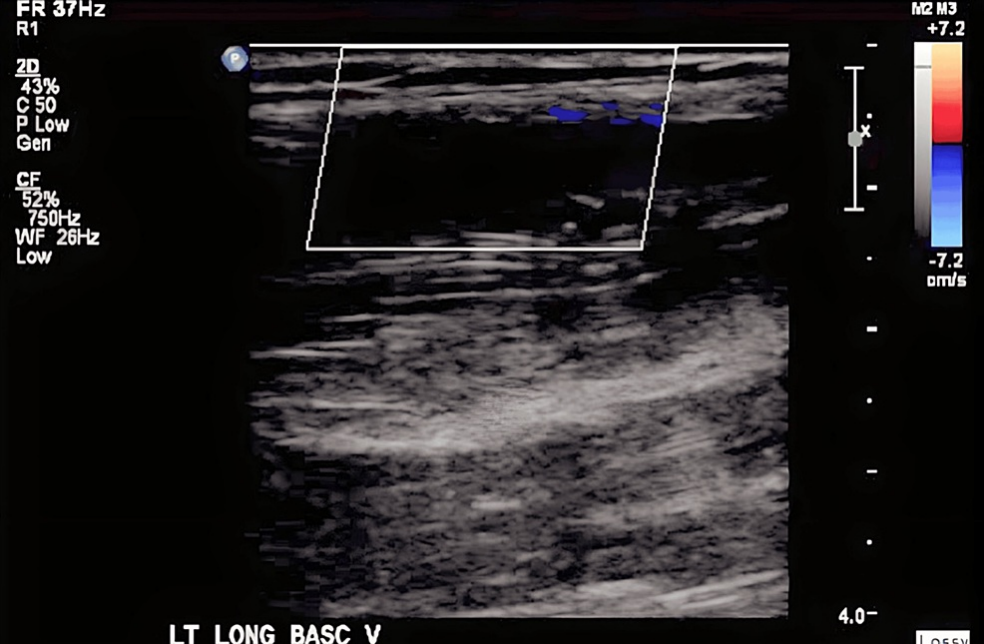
Venous Doppler showing thrombosis in the left long basilic vein
